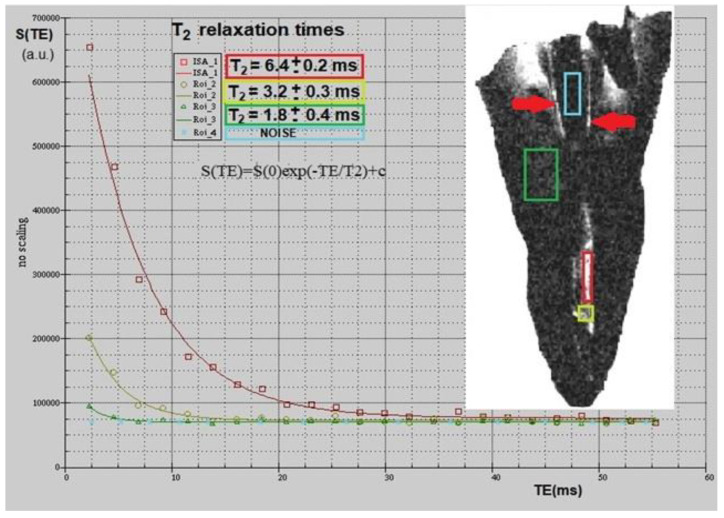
T2-weighted mMRI of tooth’s transverse section (white insert) with the different ROIs from which the data were extracted: light blue, Gutta-percha; green, dentine; red and yellow, voids and underfilled canals. The graph shows the experimental data decay vs. echo time (TE) in different tooth ROIs and the resulting fit function. From the fit of the function to data, T2 values were obtained in different regions of the tooth. Red arrows indicate spaces filled with water between dentine and Gutta-percha.
